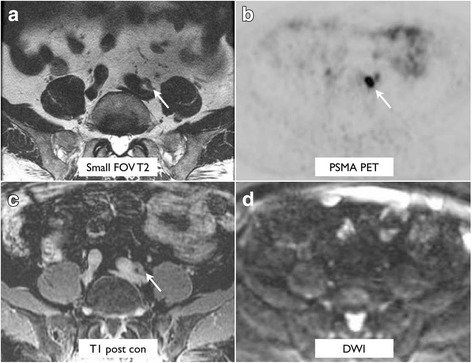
A 70-year-old man with biochemical recurrence (PSA of 10.5), who had a left common iliac lymph node measuring 1.3 × 0.6 cm seen on T2-weighted imaging (a), PSMA PET (b), and post-gadolinium T1-weighted imaging (c). The lymph node was not seen on diffusion-weighted imaging (d)
